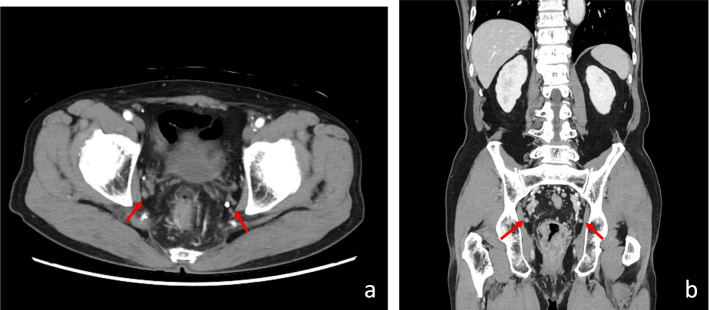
CT examination showed pelvic lymph node swelling in the axial plane (a) and the coronal plane (b) (red arrowhead)
